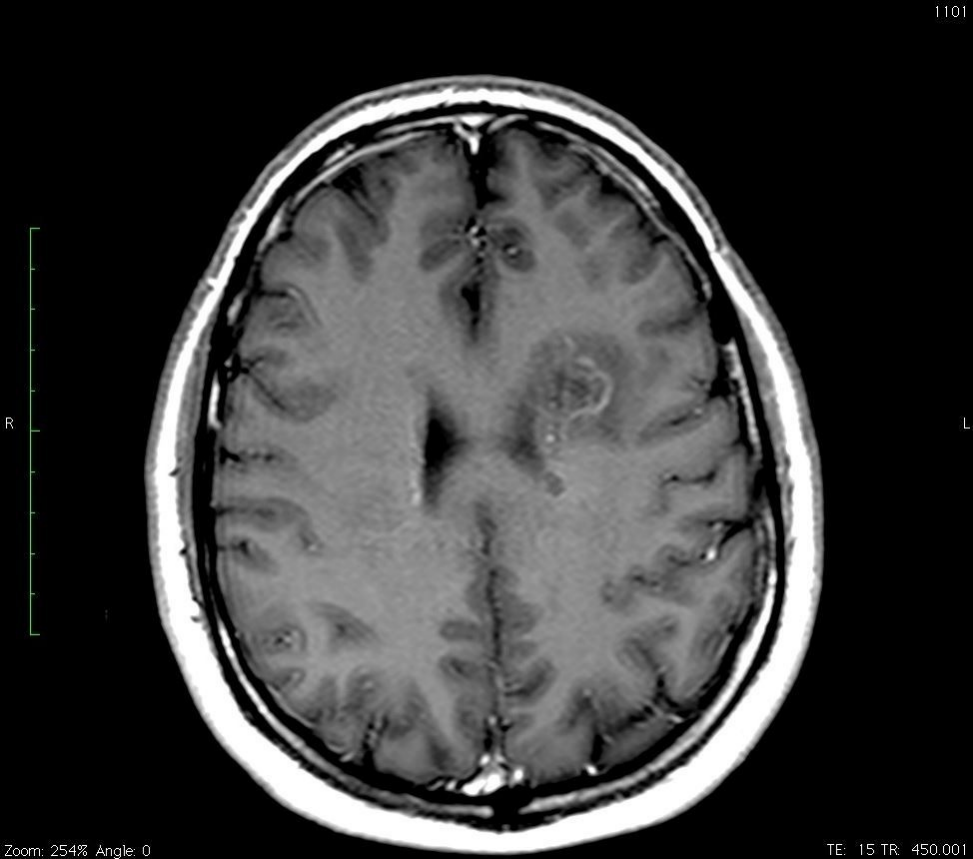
T1 MRI post contrast with a contrast-enhancing lesion with the “Open Ring” appearance.
